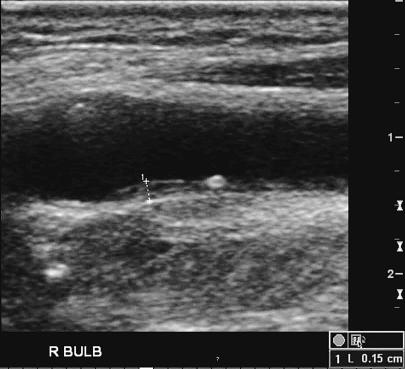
Duplex Ultrasound of the Right Carotid Artery The image shows diffuse thickening of the intimal wall (intimal medial thickness of 0.15 cm at right bulb, 0.16 cm at left bulb) with focal plaques present at bilateral distal common carotid arteries at bulb regions.
